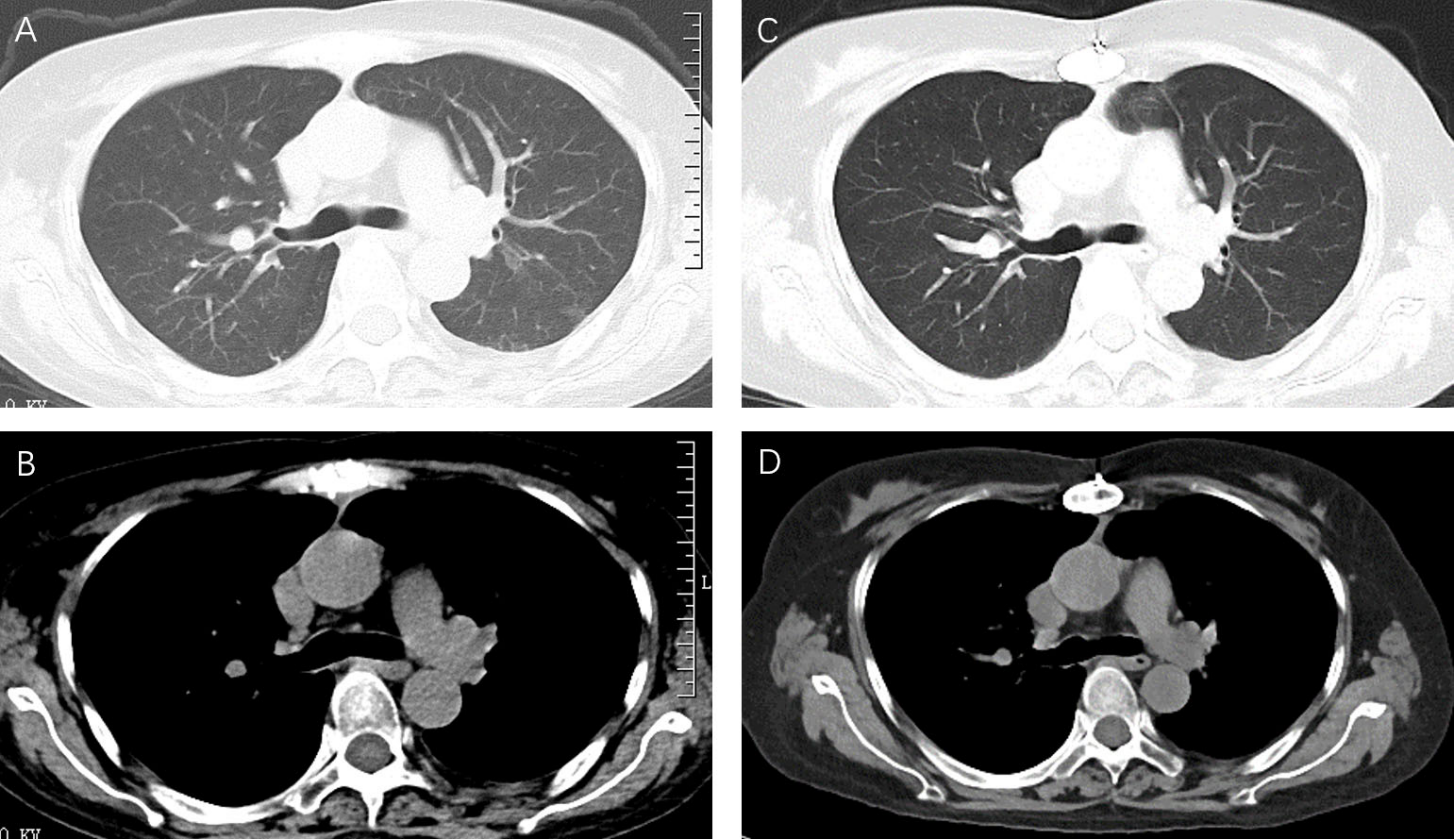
A reexamination of chest CT after 2 months of amphotericin B and fluconazole therapy revealed no pleural effusion (A, B). Follow-up chest CT showed that the patient was still free of pleural effusion 1 year later (C, D).
